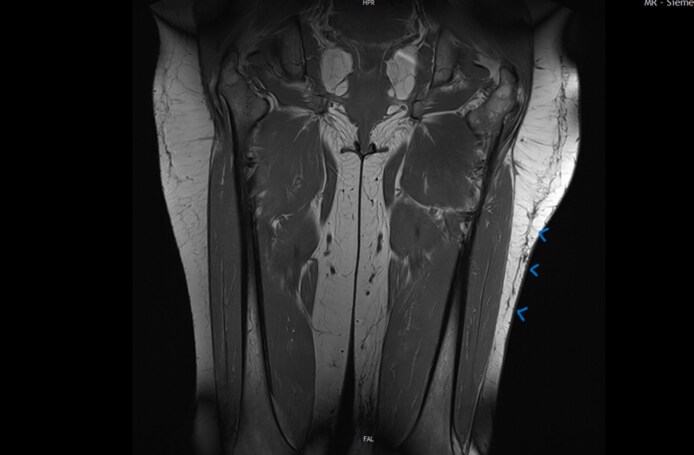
Follow-up MRI conducted 3 months after the second water jet–assisted liposuction of the left thigh. Arrows mark the marginal subcutaneous cleft that remains visible in the MRI image.
